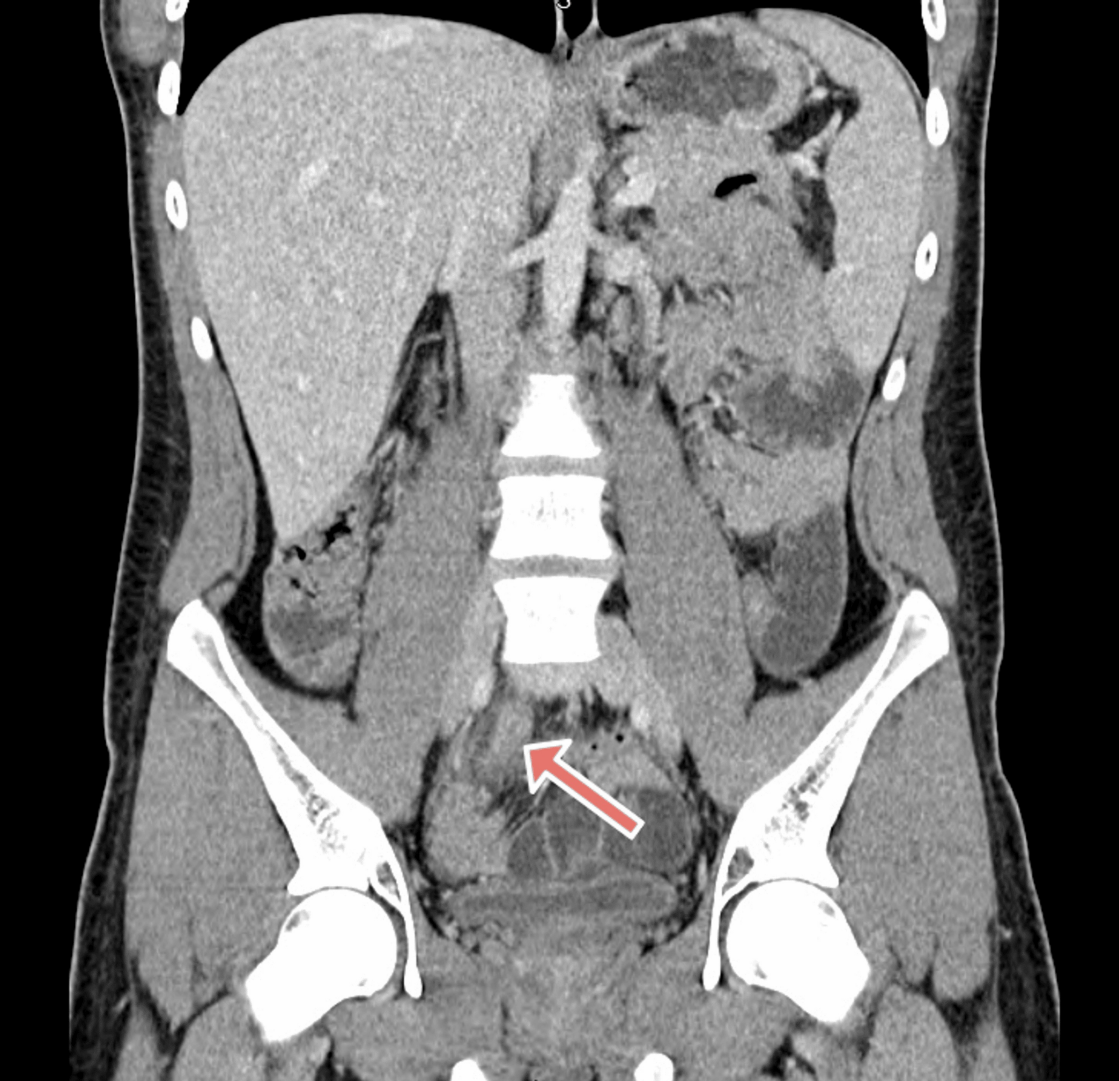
Coronal CT of the abdomen and pelvis with contrast showing appendicitis
